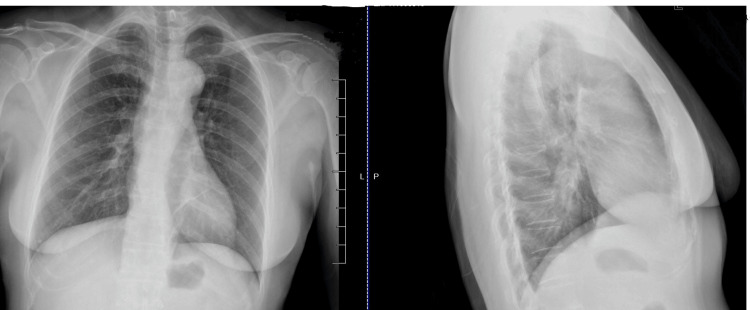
Chest X-rays in PA and lateral view showed no infiltrate, no tumor, or no scar.
